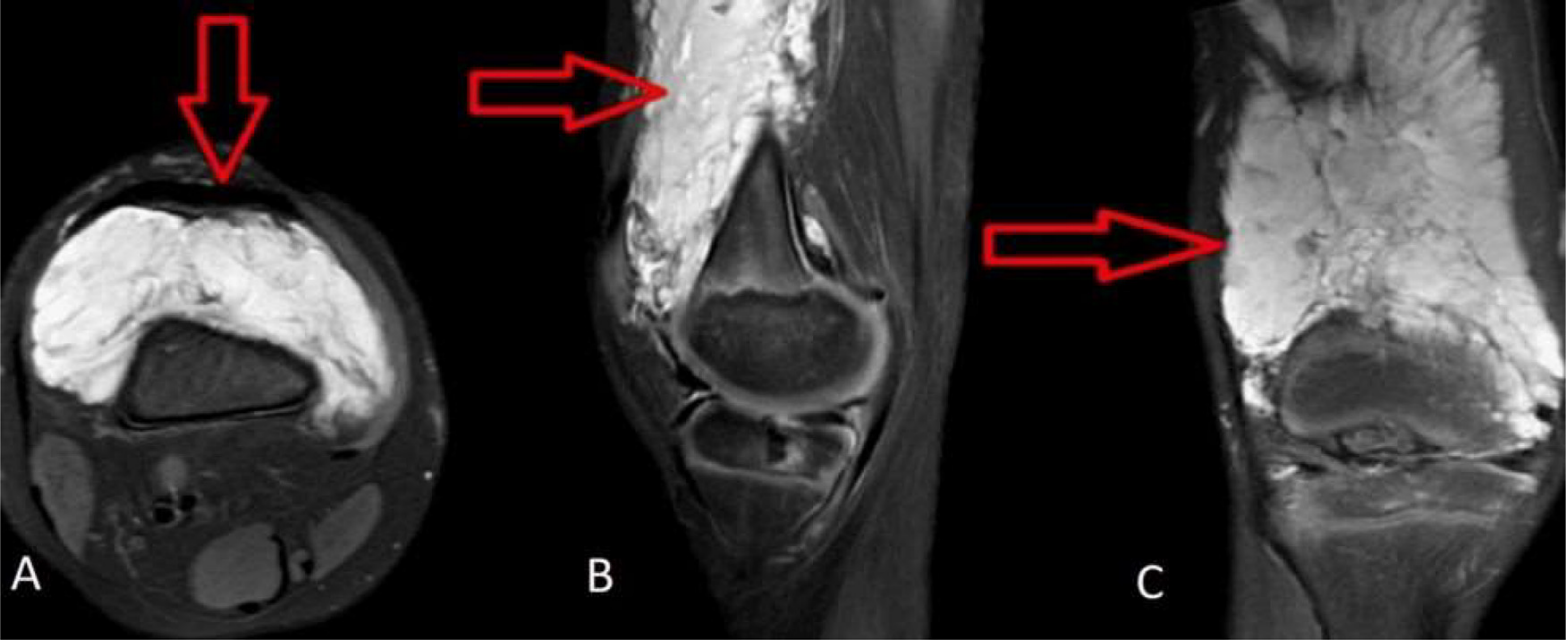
Magnetic Resonance Imaging (MRI) showing multiple, dilated, tortuous serpentine Proton Density Fat Saturated (PDFS) hyperintensity along vastus medialis muscle with hypertrophy of the muscle (red arrow). A-MRI PDFS axial section, B-MRI PDFS sagittal section C-MRI PDFS coronal section.
